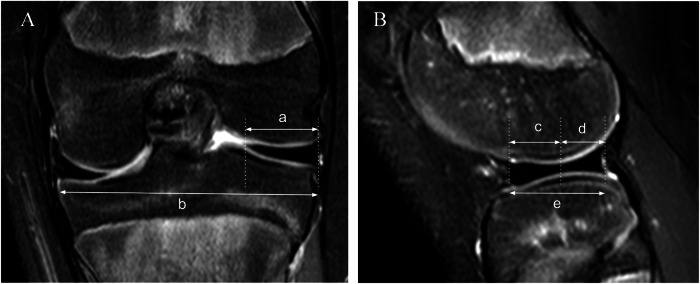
Images of MRI measurements obtained from a child presenting with an incomplete DLM. A Coronal slice. Meniscal width (a) and maximum tibial width (b). The RMT to the tibia: a/b x 100%. B Sagittal slice. Width of the anterior and posterior horns (c and d, respectively) to the maximum meniscal diameter (e). Percent coverage of the meniscus: (c + d)/e × 100%
